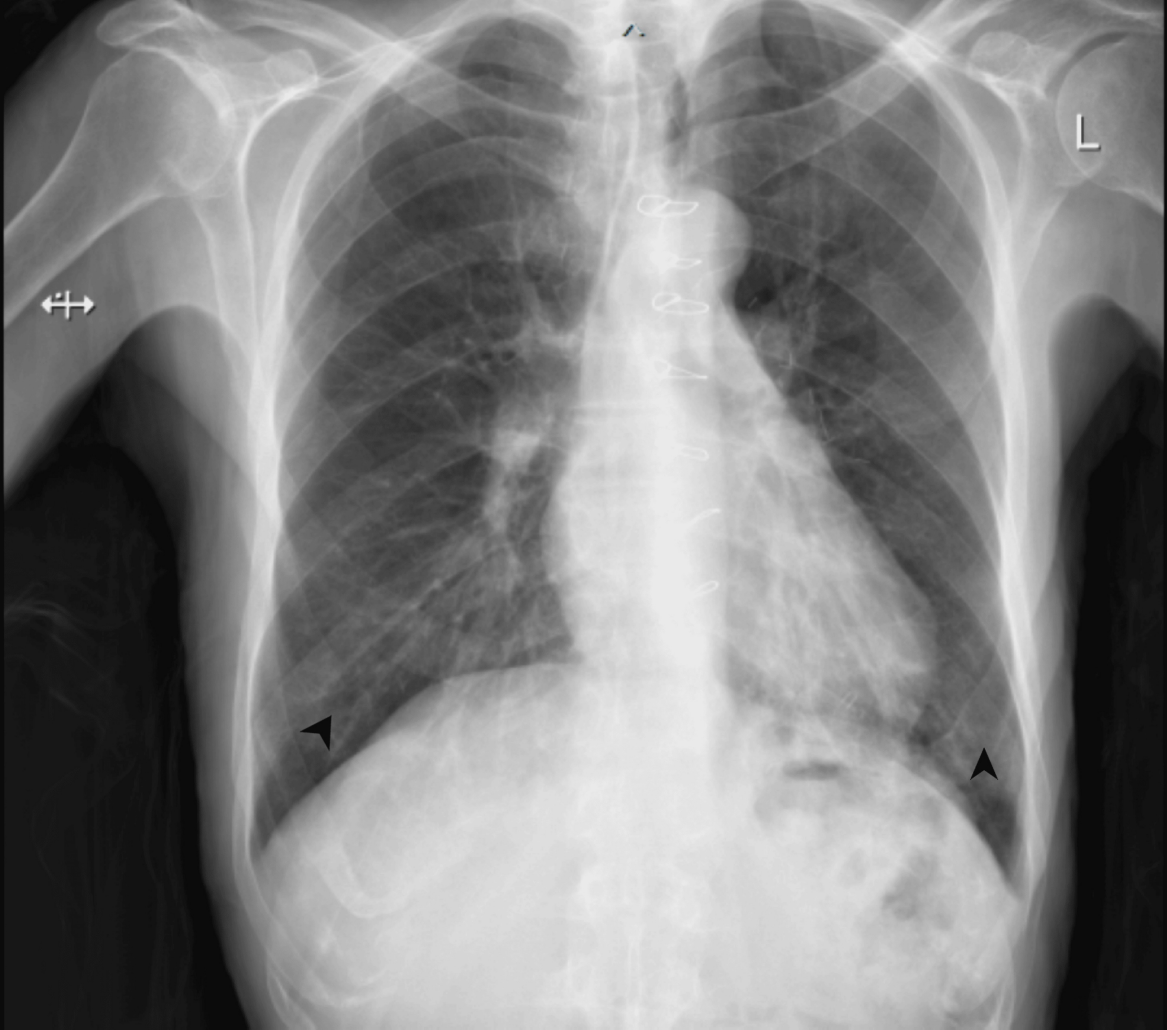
Chest X-ray upon presentation showing bilateral lower zones infiltrate indicated by the arrows.
